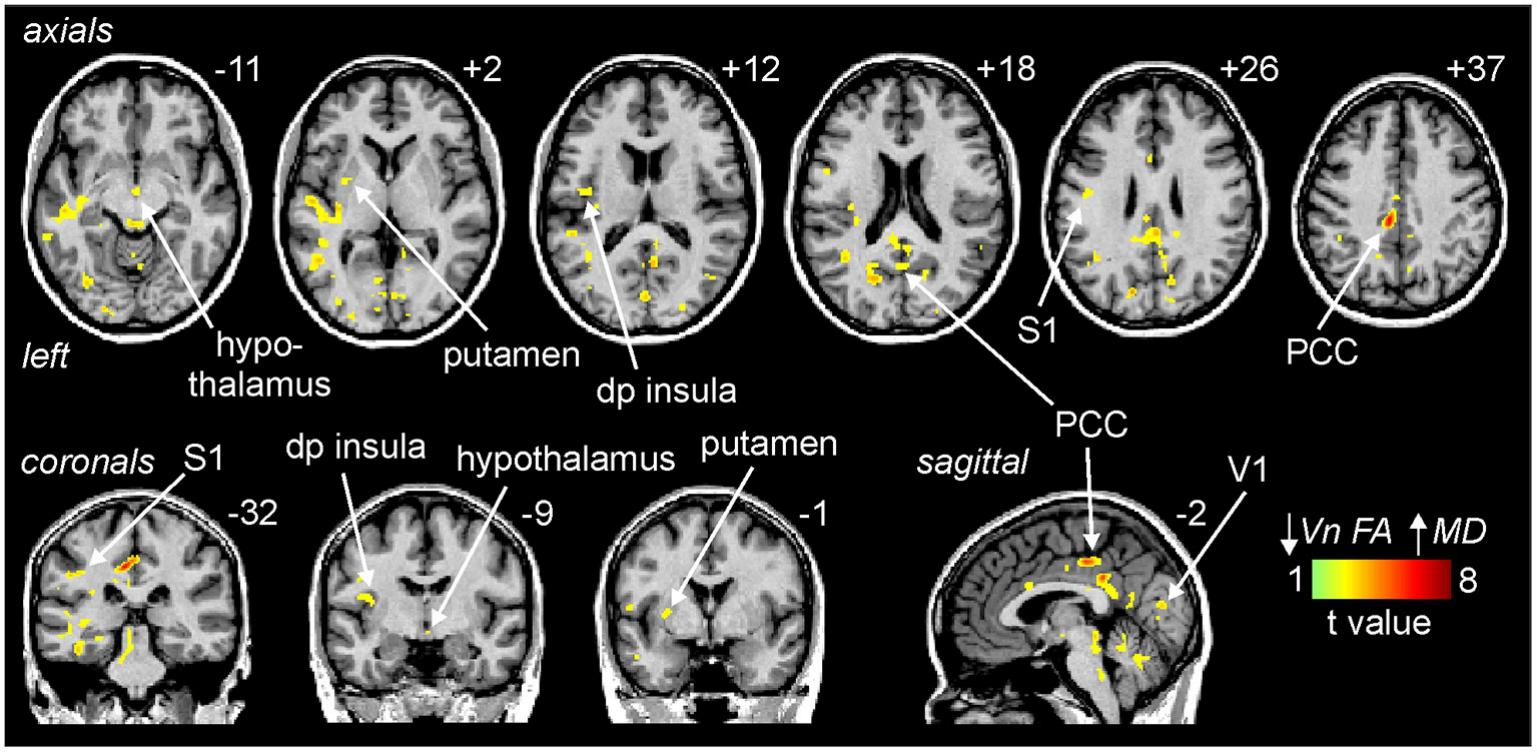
Areas above the brainstem in which reductions in trigeminal nerve fractional anisotropy (FA) were significantly correlated with increases in above the brainstem mean diffusivity (MD) assessed in migraine (*n* = 38) and control participants (*n* = 38). Significant clusters are overlaid onto a mean T1-weighted anatomical image. Slice locations in Montreal Neurological Institute (MNI) space are indicated to the top right of each slice. Note that MD was significantly correlated to nerve FA in the posterior cingulate cortex (PCC), primary somatosensory cortex (S1), dorsoposterior insula (dp insula), hypothalamus, putamen and the primary visual cortex (V1).
